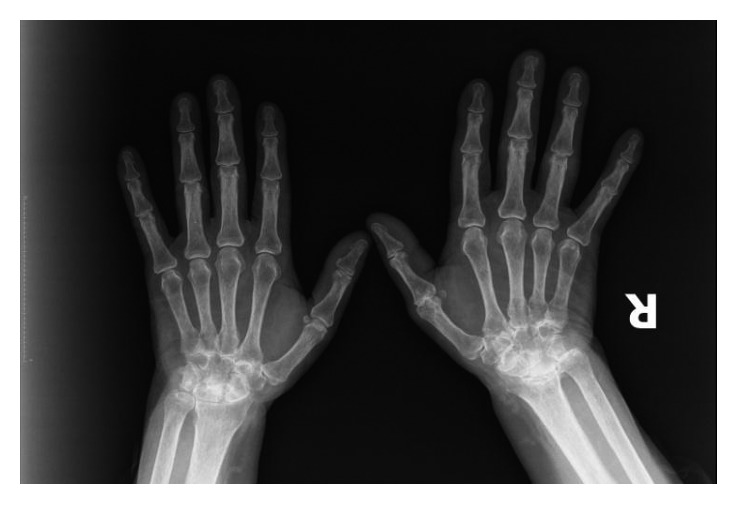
X-ray findings of the hands (patient 3).
